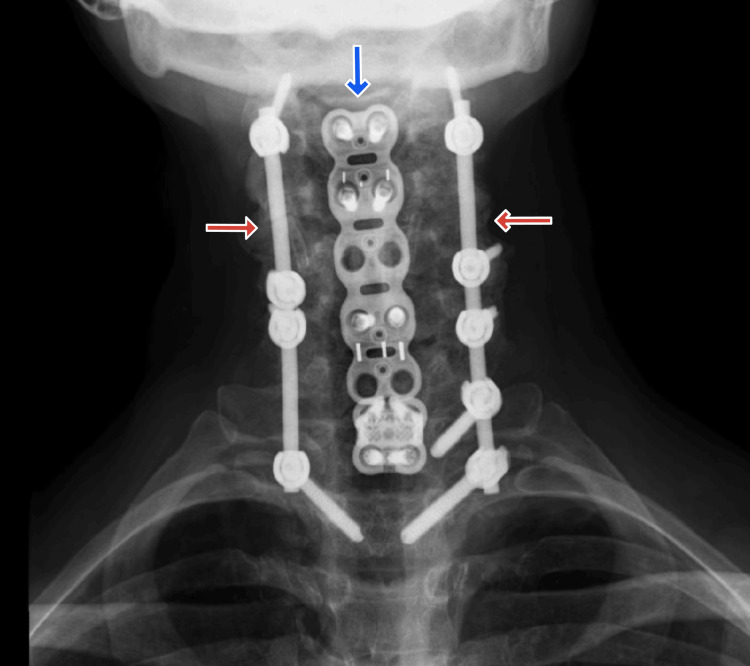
Posterior-anterior radiograph of cervical spine Posterior fixation with bilateral pedicle screws and segmental spine plating can be seen from C2-T2 levels, marked by red arrows. Anterior cervical discectomy and fusion with plate extends from C3-T1, marked by the blue arrow. Obtaining this image allows the interventional pain physician to assess the feasibility of lead placement in the goal distribution.
